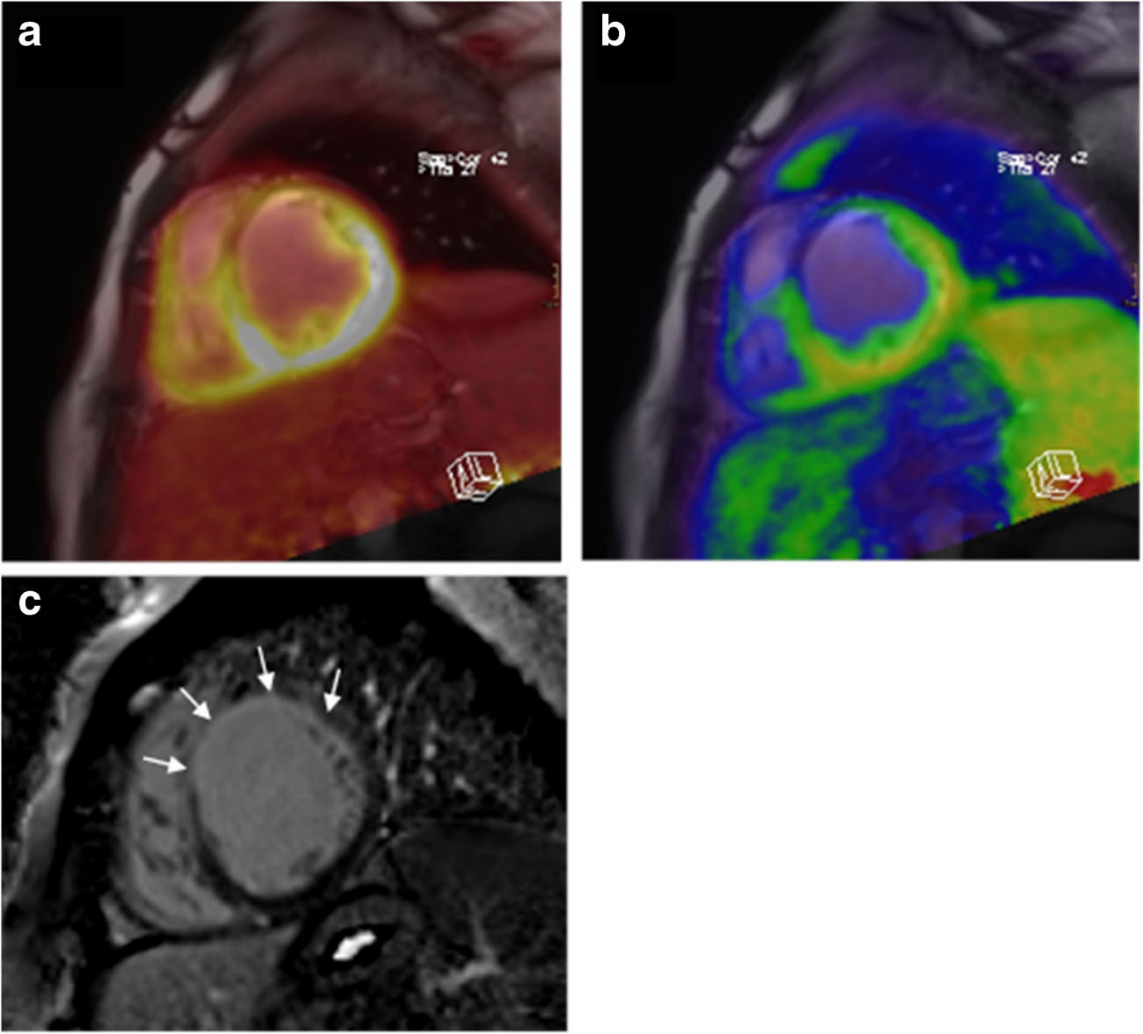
Cardiac PET/MRI of a 57-year-old male patient with advanced coronary artery disease and deteriorated LV function showing an agreement of missing ^18^F-FDG and ^13^N-NH3 uptakes with presence of transmural late gadolinium enhancement in the anterior wall of the myocardium, which was rated as transmural scar. (A) Image fusion of ^18^F-FDG PET and LGE MRI. (B) Image fusion of ^13^N-NH3 PET and LGE MRI. (C) Extent and transmurality of scar determined by MRI LGE
